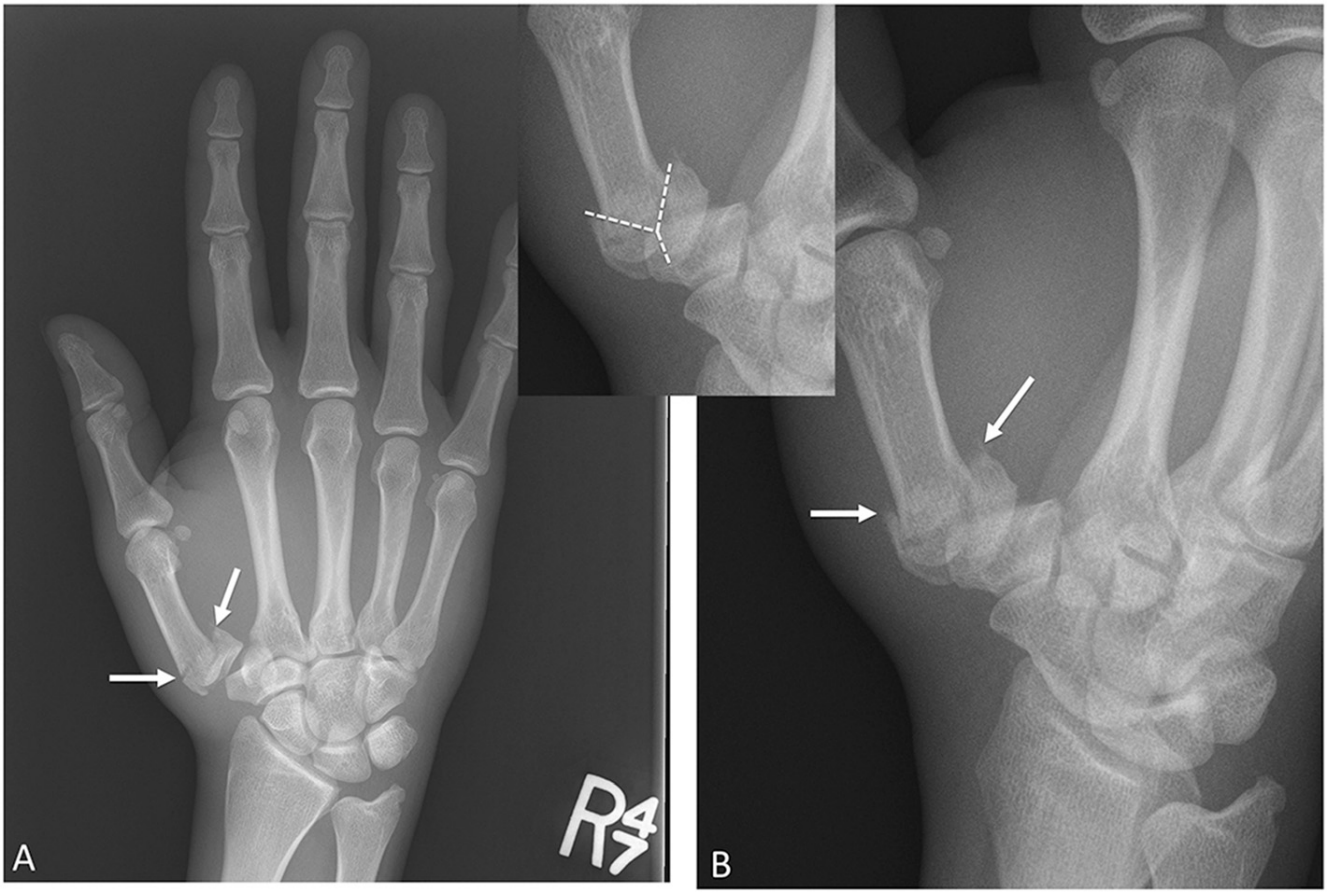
Rolando fracture. AP (a) and oblique radiographs (b). Comminuted fracture at the base of the first metacarpal (*arrows*), with intra-articular extension into the first carpal-metacarpal joint. *Inset* picture delineating the typical “Y” shaped fracture pattern. These fracture patterns can also be “T” shaped
